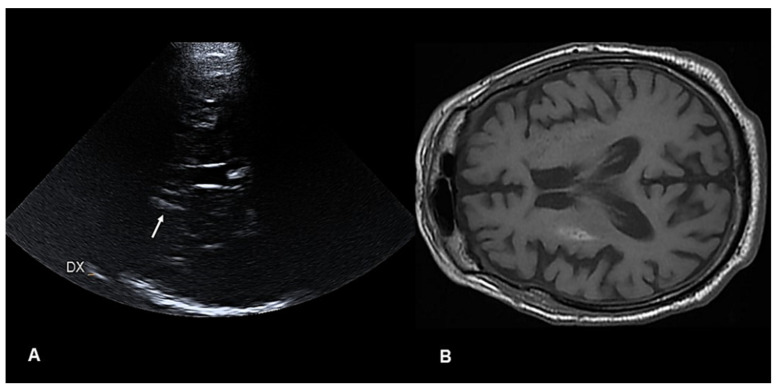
(A) (*left panel*) Parenchymal transcranial sonography showing hyperechogenicity in the right (DX) lenticular nucleus (*white arrow*); (B) (*right panel*) axial brain magnetic resonance imaging showing a T1-weighted hyperintensity in the right putamen.
